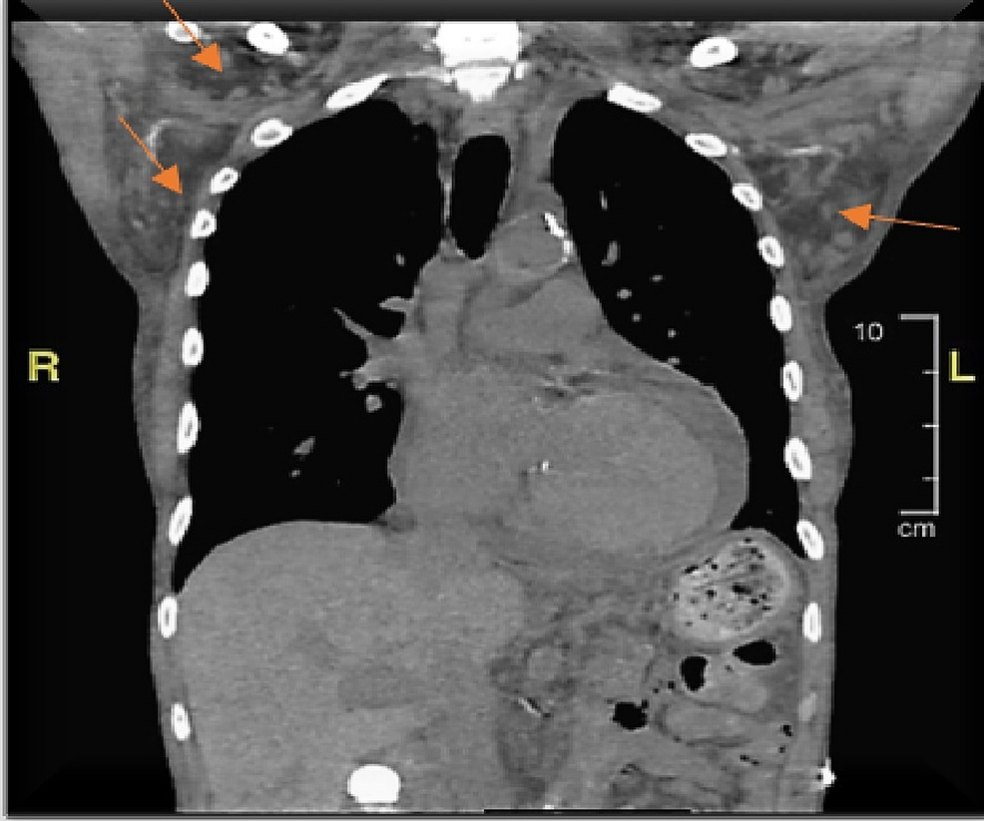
CT chest with fluid in the soft tissue of the axilla and chest wall.
